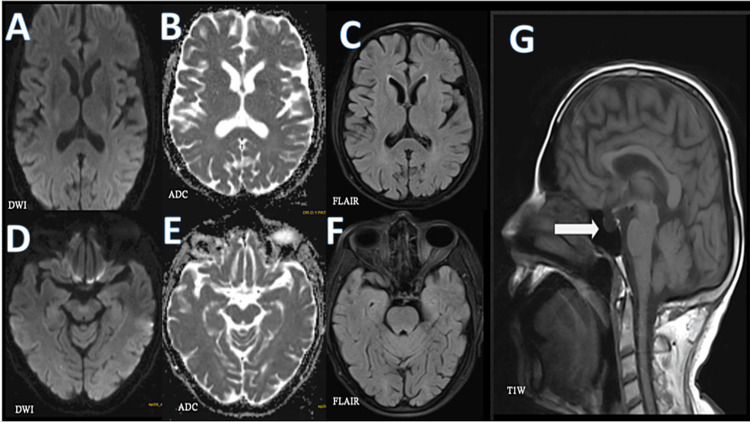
MRI brain revealed an empty sella sign Sections at the level of basal ganglia (A,B,C) and sections at the level of the midbrain (D,E,F) demonstrate corresponding DWI, ADC, and FLAIR images, which show no infracts or lesions. The T1W (G) sagittal image revealed an empty sella sign (marked by a white arrow). ADC, apparent diffusion coefficient; DWI, diffusion-weighted imaging; FLAIR, fluid-attenuated inversion recovery; T1W, T1-weighted
